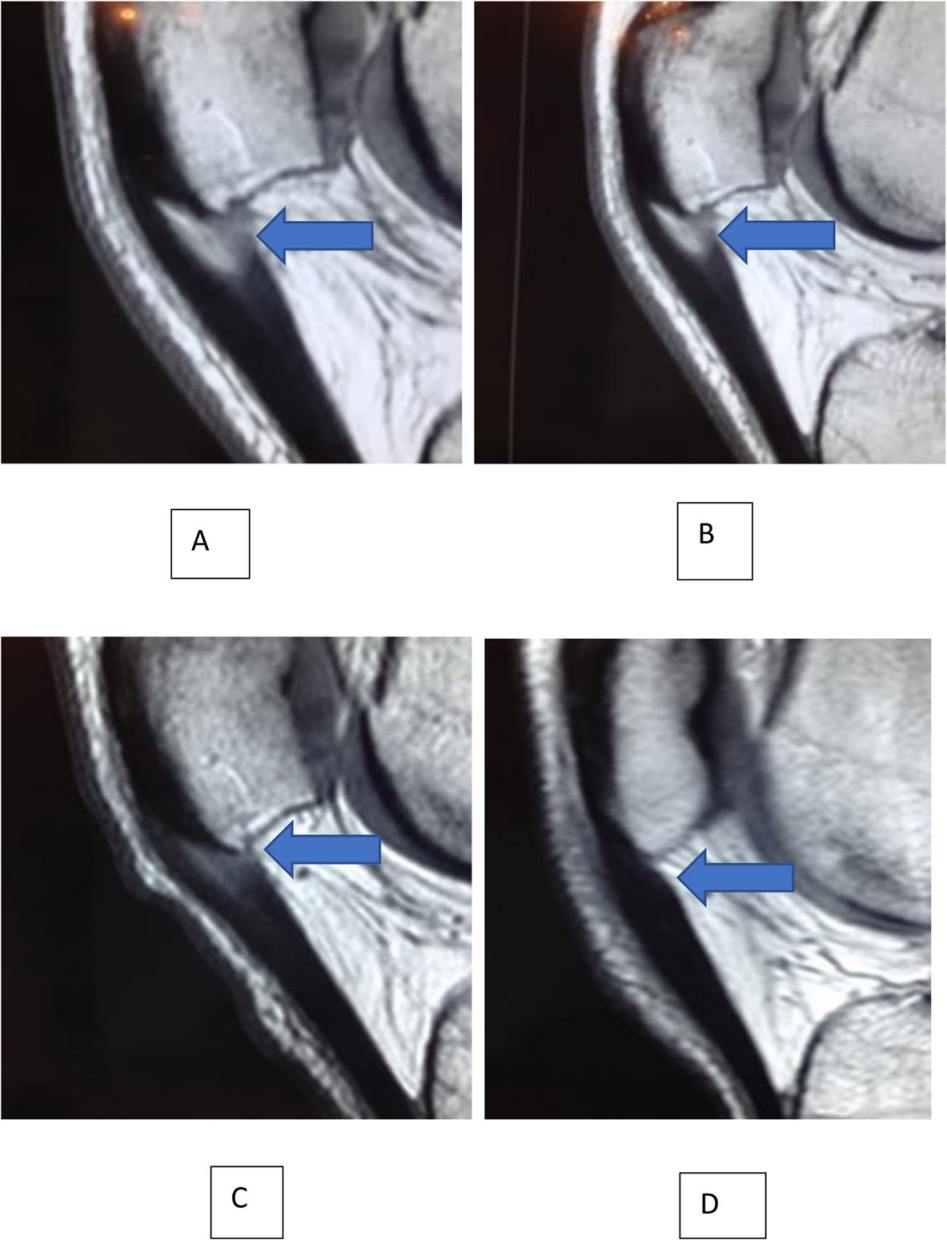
Sagittal T1 MR images of insertional tendinopathy healing at (A) Baseline, (B), 3, (C), 6, and (D), 12 months post-injections. Note the decreasing area of tendinosis\Tear (Blue Arrows). Image D: Only a thickening of the tendon can be observed
